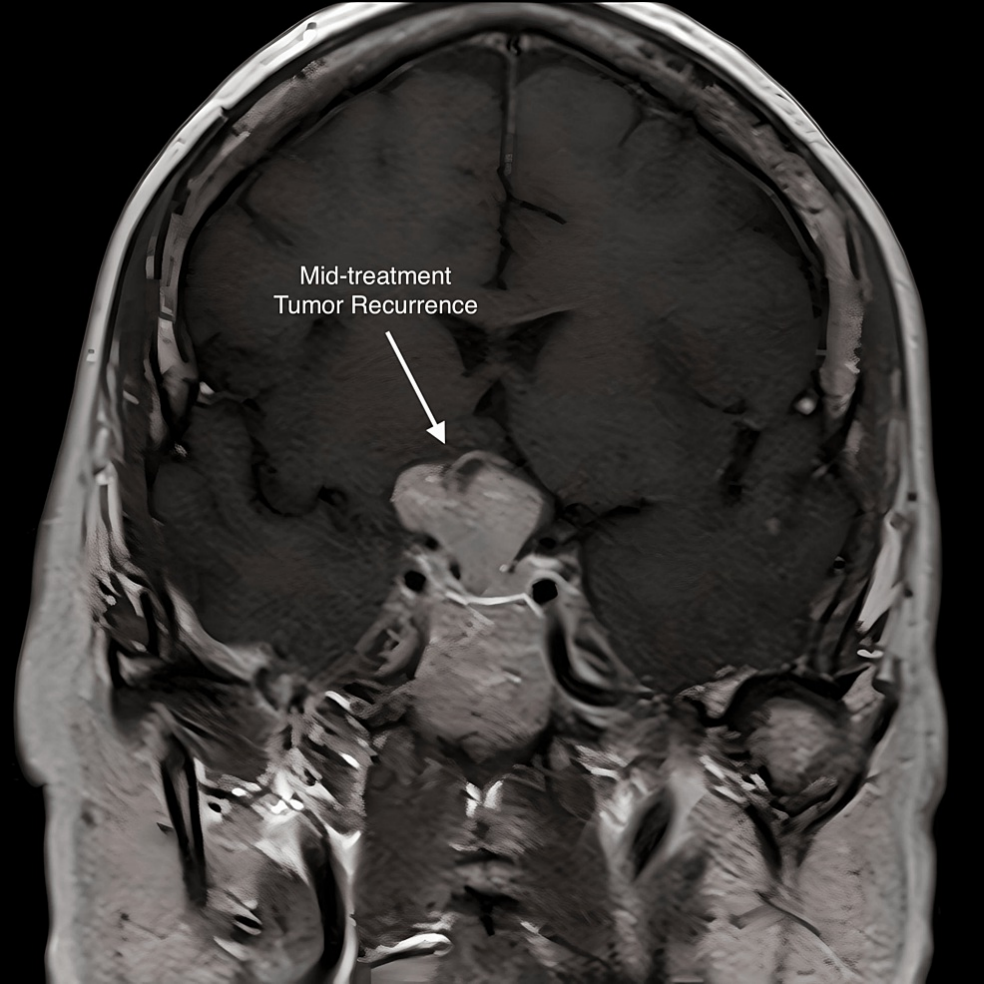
Post-operative mid-coronal MRI with contrast; this shows a 2.6 cm x 2.4 cm x 2 cm craniopharyngioma with substantial interval progression with a persistent anterior cystic component causing mass effect
